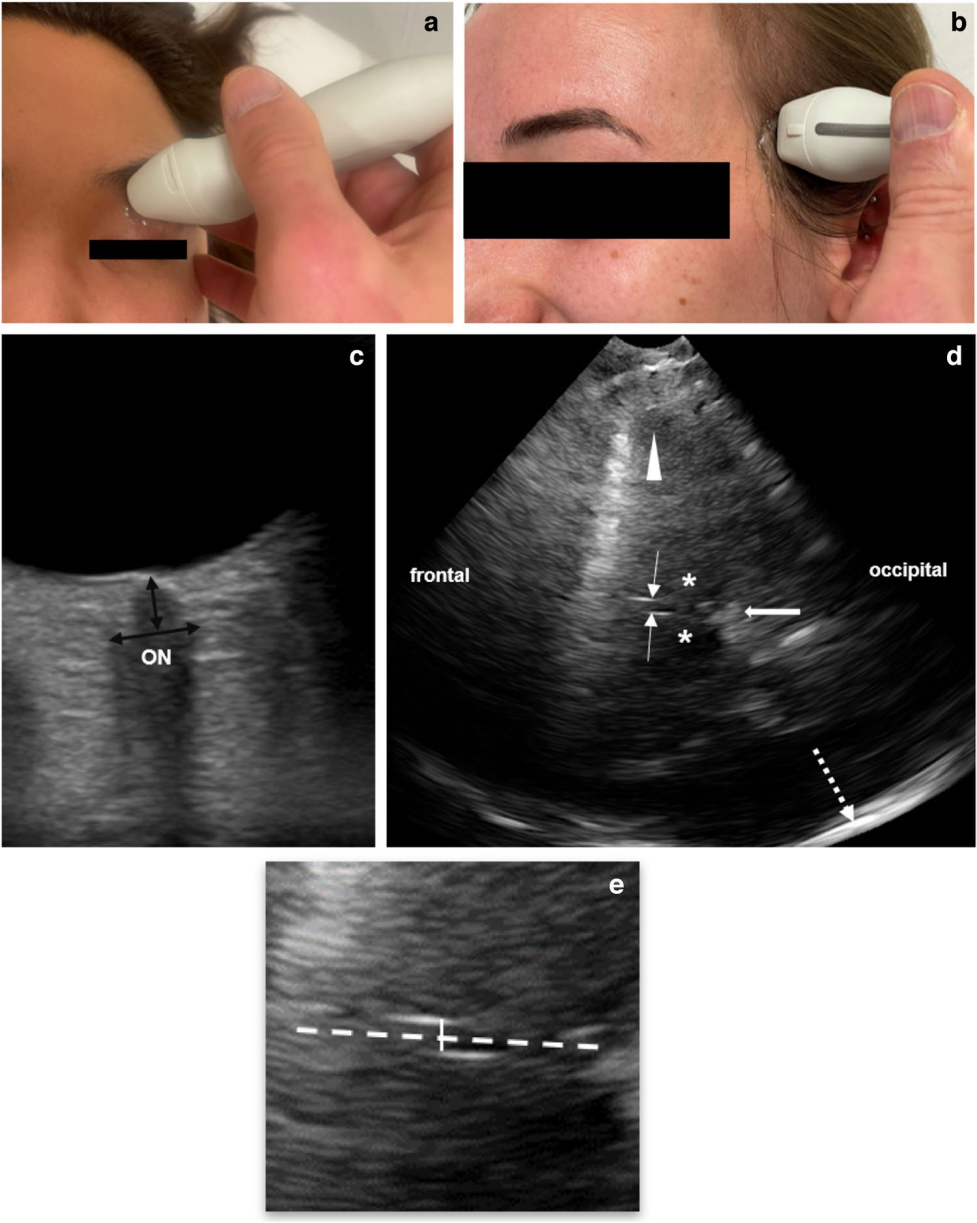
B-scan ultrasound (US) of the optic nerve sheath and third ventricle diameters in a 13-year-old girl from the control group. a For transorbital US examination, a 12-Mhz linear transducer is placed axially on the closed eyelid. The examiner’s hand can be placed lightly on the side of the patient’s head to improve stability. b For transtemporal US examination, a 1–4-MHz phased-array transducer is placed axially and pre-auricularly between the lateral canthus and the upper border of the ear. The probe orientation marker on the side of the probe should be oriented forward so that the frontal intracranial structures appear on the left side of the monitor. c Transorbital US of the optic nerve sheath diameter (4.71 mm) with exact region of optic nerve sheath diameter measurement (*arrows*). The optic nerve (*ON*) appears hypoechogenic. d Transtemporal US of the third ventricle diameter (3.1 mm), showing hyperechogenic walls of the third ventricle (*thin arrows*), bilateral, hypoechogenic-appearing thalami (*asterisks*), hyperechogenic-appearing pineal gland (*thick arrow*), ipsilateral brain parenchyma (*arrowhead*), and contralateral skull bone (*broken arrow*). e 1.5× magnification of a section of (d) showing the region of the third ventricle. Area of exact third ventricle diameter measurement (*solid line*) and the midline of the brain (*broken line*)
